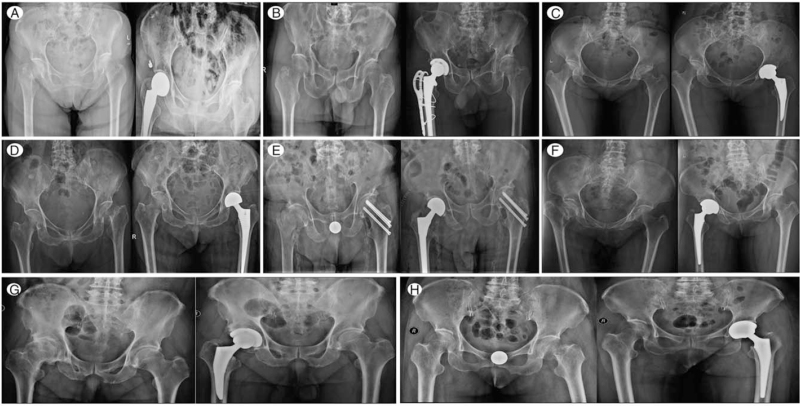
Anteroposterior (AP) pelvic radiographs of all 8 patients in the present study. (A) Case 1. AP pelvic radiographs showing a femoral neck fracture and postoperative radiograph. (B) Case 2. Preoperative and postoperative AP radiographs showing an intertrochanteric fracture. (C and D) Case 3 and 4. Preoperative and postoperative AP radiographs showing a femoral neck fracture. (E) Case 5. Preoperative pelvic radiographs showing an intertrochanteric fracture and postoperative radiograph. (F) Case 6. Preoperative and postoperative radiographs showing a femoral neck fracture. (G) Case 7. Preoperative and postoperative radiographs showing an osteonecrosis of the femoral head. (H) Case 8. Preoperative and postoperative radiographs showing a femoral neck fracture.
